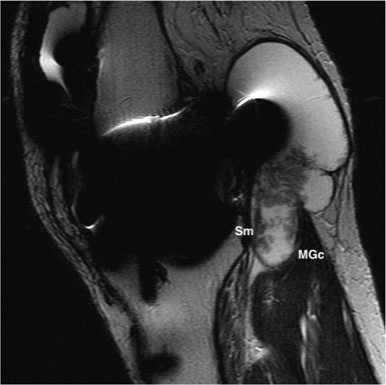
Heterogeneous popliteal cyst in an 89-year-old woman with known total knee arthroplasty presenting with a palpable mass. Sagittal T2-weighted MRI shows a few septa and hypointense internal debris in an otherwise common Baker’s cyst. Despite the severity of artifact due to metallic hardware, it is still possible to appreciate its relationship to the medial gastrocnemius and the semimembranosus tendons. Absence of enhancement after gadolinium intravenous administration was confirmed in the same study (not shown). MGc, medial gastrocnemius; Sm, semimembranosus
